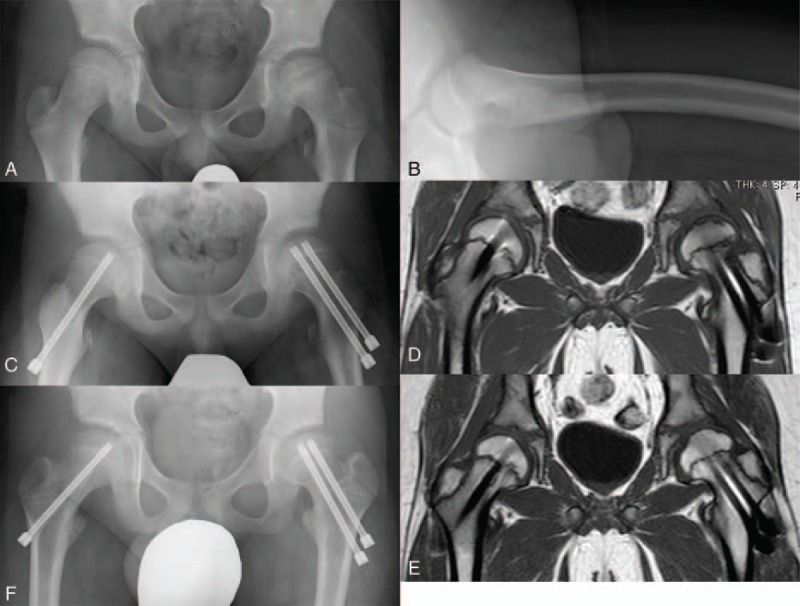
Anteroposterior (A) and Lauenstein (B) hip radiographs of case 10 demonstrating posteriorly displaced femoral epiphysis with the HSA of 141 degrees and the PTA of 35 degrees. Postoperative anteroposterior hip radiograph showing reduced femoral epiphysis of the affected side and prophylactic pinning on the contralateral side (C). Coronal T1-weighted magnetic resonance imaging (MRI) of the hip taken at 3 months after surgery indicating linear low-intensity area at the subchondral bone region of the affected hip (D). Coronal T1-weighted MRI of the hip taken at 1 year after surgery demonstrating disappearance of the abnormal signal intensity observed in previous films (E). Anteroposterior hip radiograph (F) at the age of 10.8 years showing spherical femoral head with no evidence of collapse. HSA = head shaft angle, PTA = posterior tilting angle.
